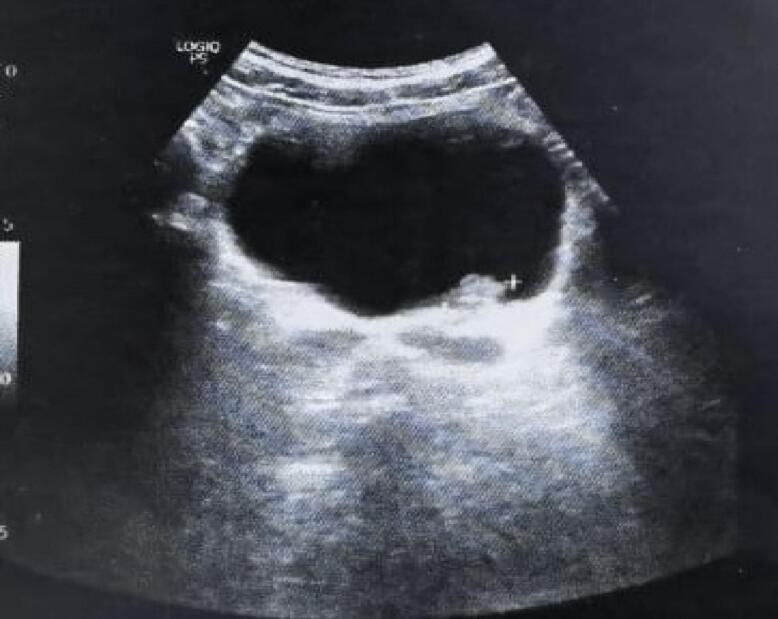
Ultrasound imaging of the bladder demonstrating intravesical polypoid masse.
